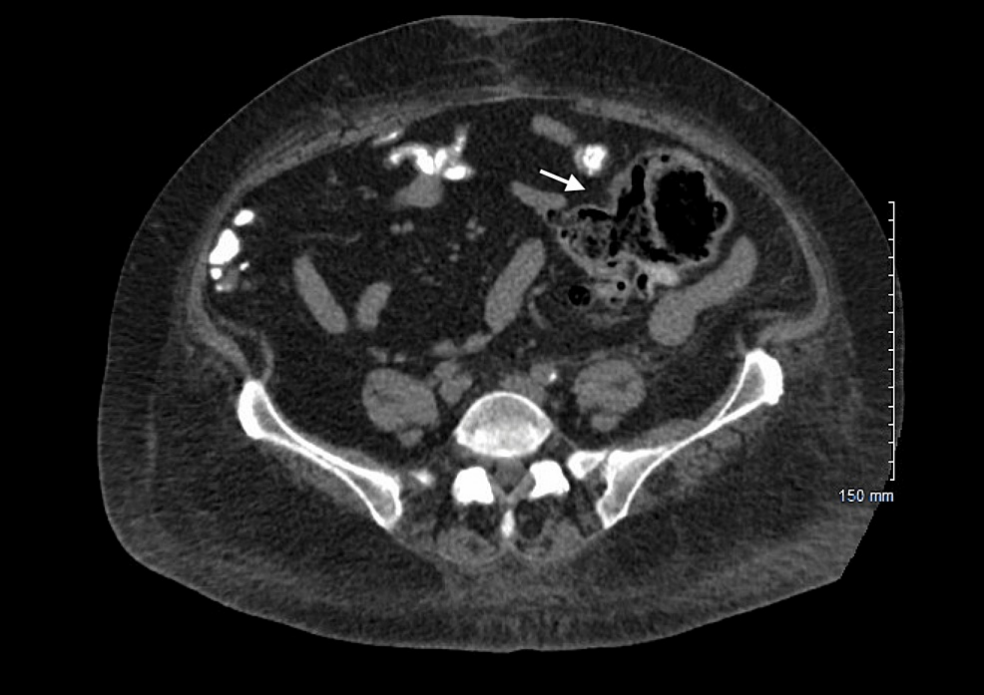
CT abdomen showing inflammatory changes in the colon. CT: computed tomography
